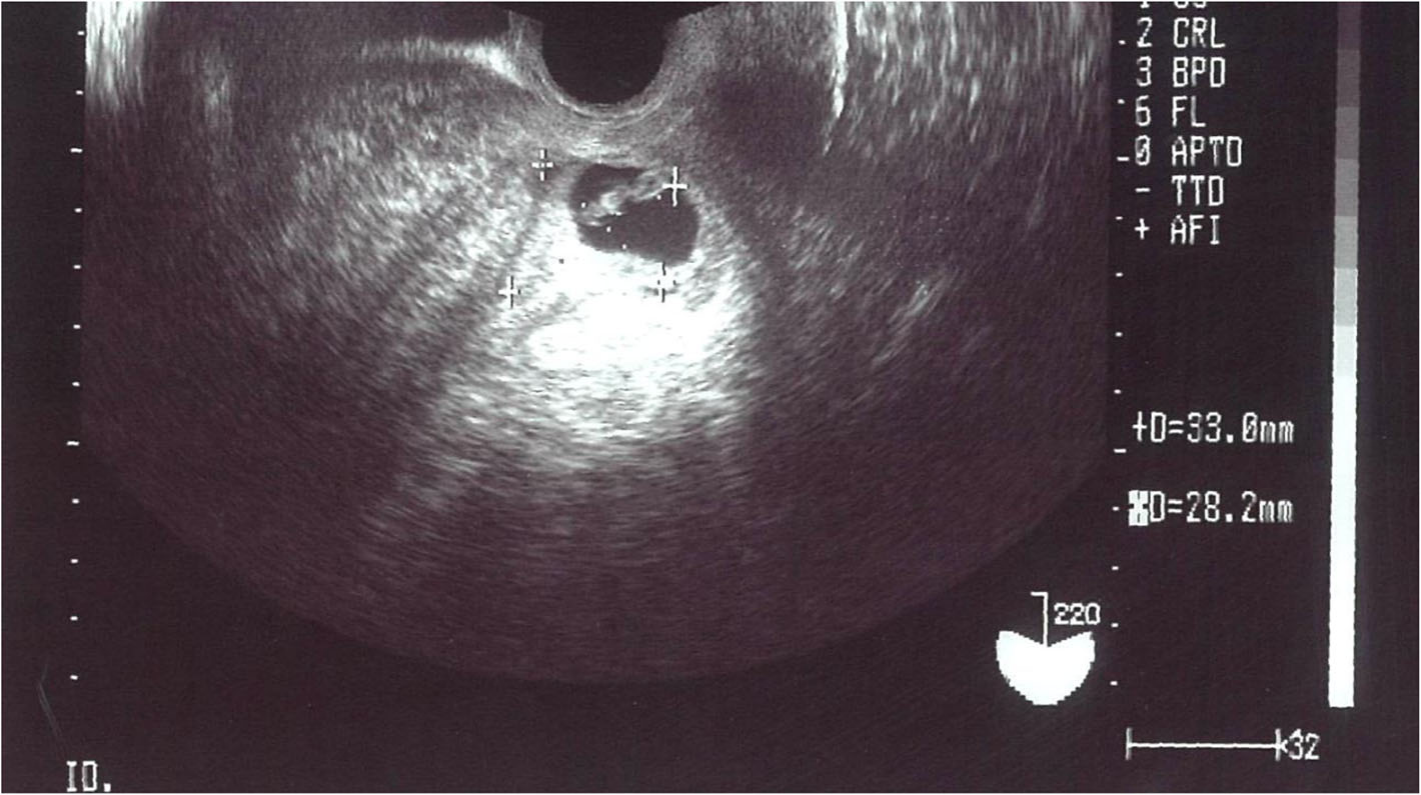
Ultrasound image of patient’s uterus at the first visit. The uterus was more than 10 cm in the thickness, and heterogeneous echogenicity could be diffusely detected throughout the myometrium. The uterine cavity was not enlarged and was rather narrow. It contained a 3 cm of gestational sac with fetal heart movement
